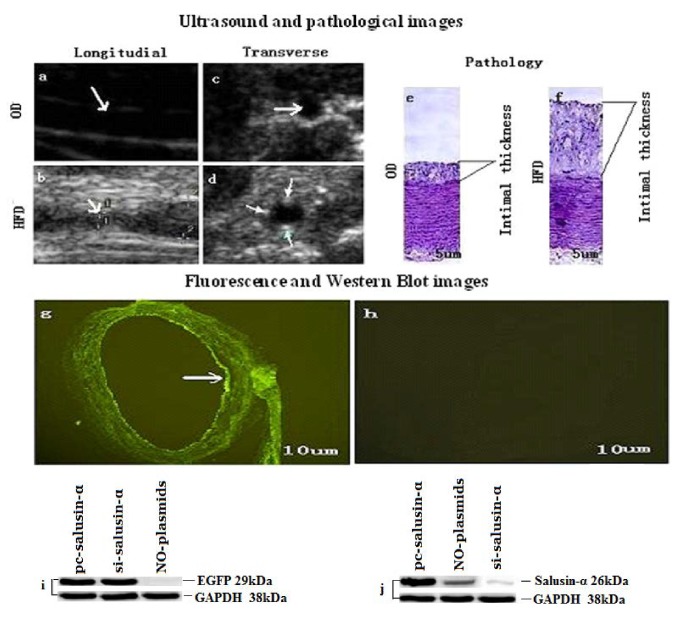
Analysis of model establishment and plasmid transfection. Representative ultrasound images (a–d) are longitudinal and transverse sections of the arteries in the HFD and OD groups (the white arrows indicate the lumen of the arteries). Representative pathological images ((e) and (f)) show the average thickness of the arterial intima in the HFD and OD groups. Representative fluorescence and western blot images (g-j) of arteries transfected by plasmids. The white arrows (g) indicate the intima transfected by the two plasmids (pc-salusin-*α* and si-salusin-*α*) with the* EGFP* reporter gene in the transfection groups, and the image in (h) indicates the arterial wall transfected by no plasmids. The western blot image (i) shows the EGFP gene expression in three different groups, which were Salusin-alpha gene overexpression (pc-salusin-*α*), salusin-alpha gene interference (si-salusin-*α*), and no plasmids transfection (NO-plasmid) group. Similarly, the salusin-alpha gene expression in the three groups was displayed by (j). The data are representative of three independent experiments (n = 3).
